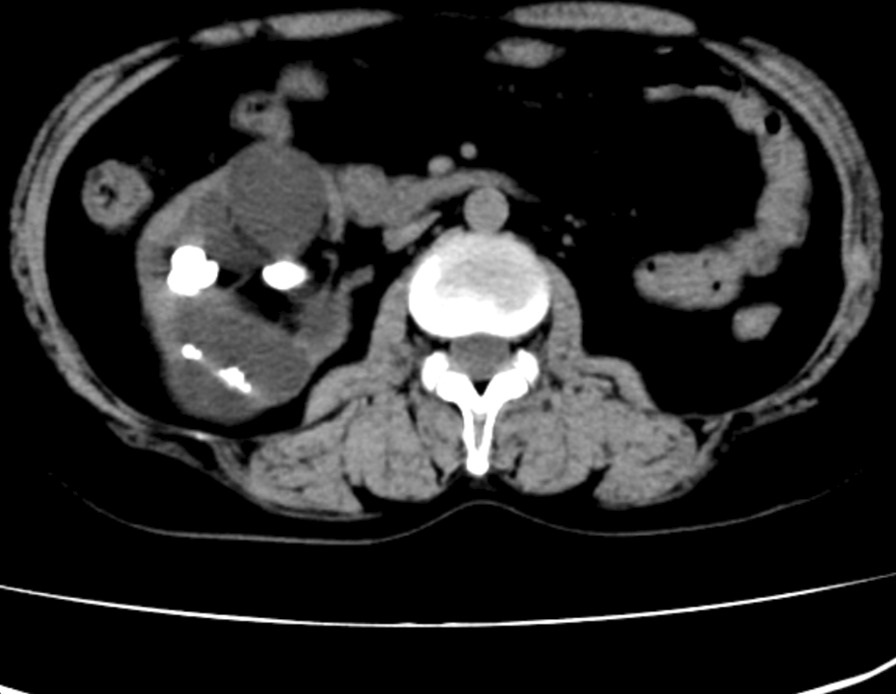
Preoperative CT scan demonstrated extremely severe hydronephrosis and multiple right renal calculi
